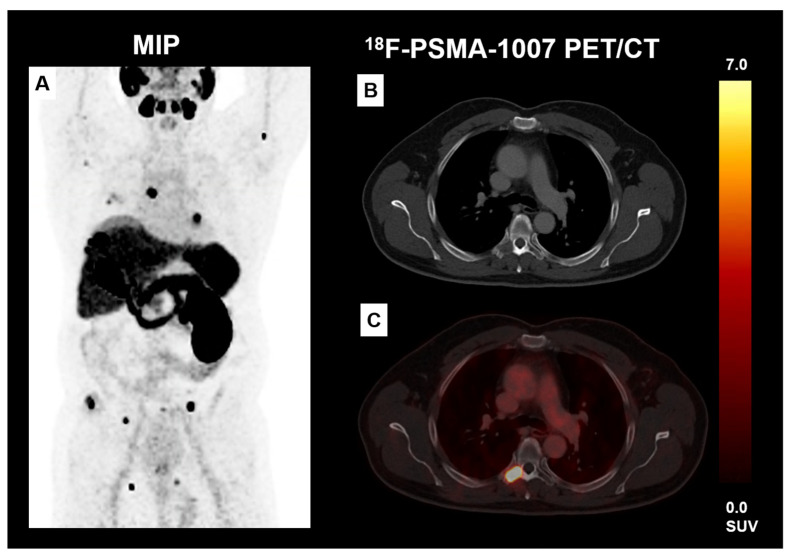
Patient with ccRCC who underwent ^18^F-PSMA-1007 PET/CT. The maximum-intensity projection (MIP) shows multifocal PSMA-positive tumoral lesions (A). Exemplarily PSMA-positive osteolytic lesion in the thoracic spine (B,C).
